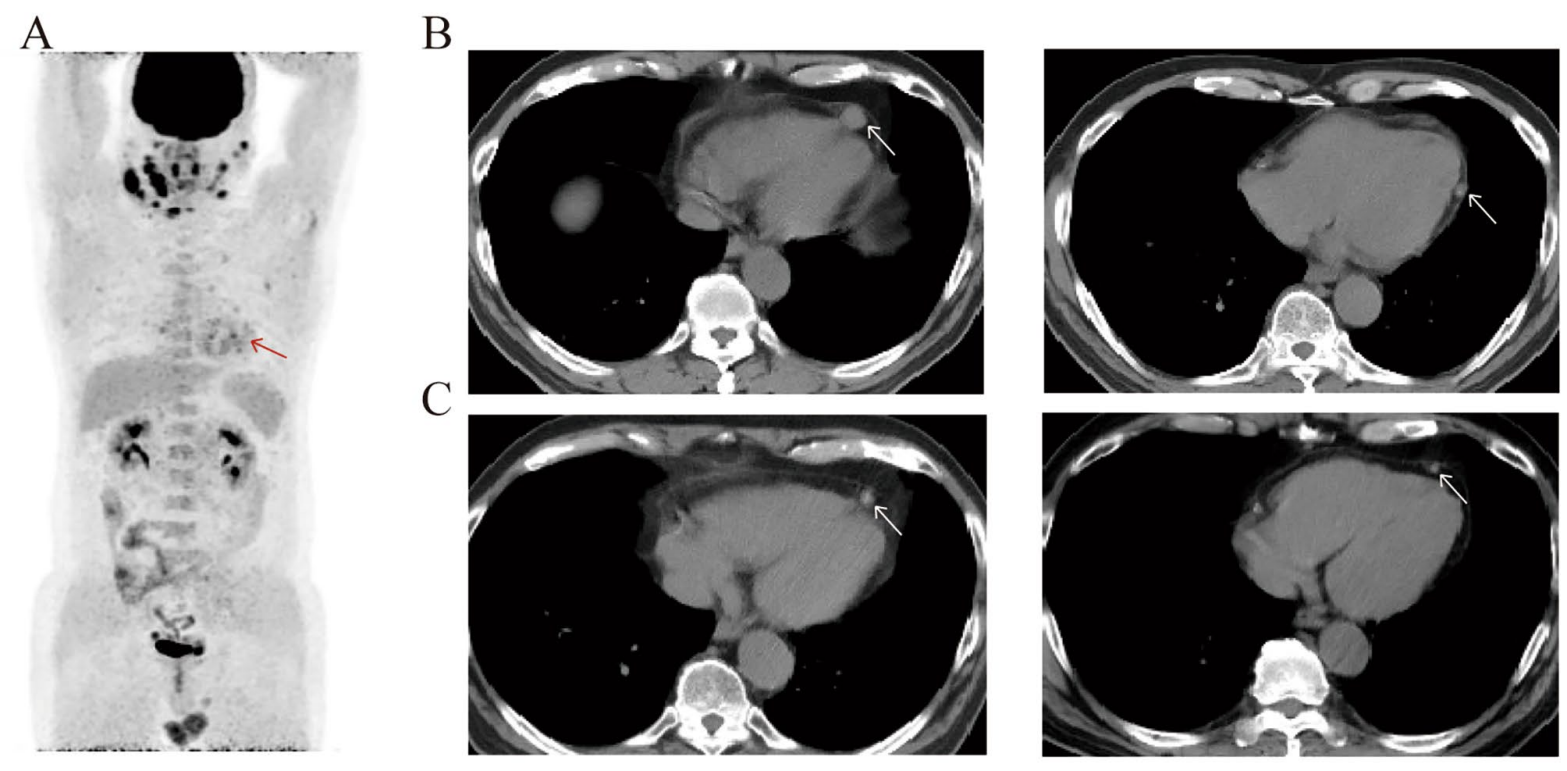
(A) PET/CT revealed multiple elevated radioactivity uptake in the aortic root wall, the left ventricular chamber, and the pericardial fat sac, SUVmax 5.3 (red arrow). (B-C) In another patient, chest CT showed multiple nodules (white arrow) of the pericardium which markedly downsized after 1 year of treatment
